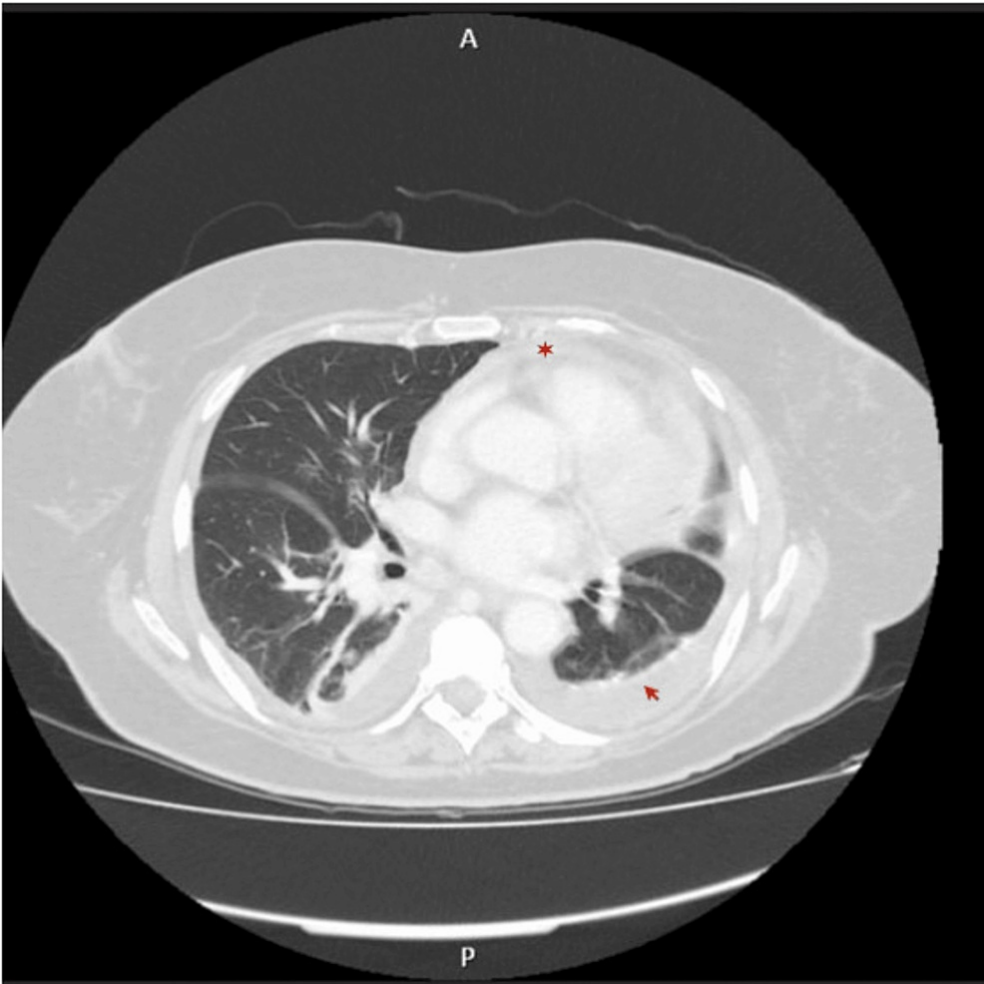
Axial computed tomography (CT) showing mild pericardial effusion (red star) and confirming the pleural effusion (red arrow)
